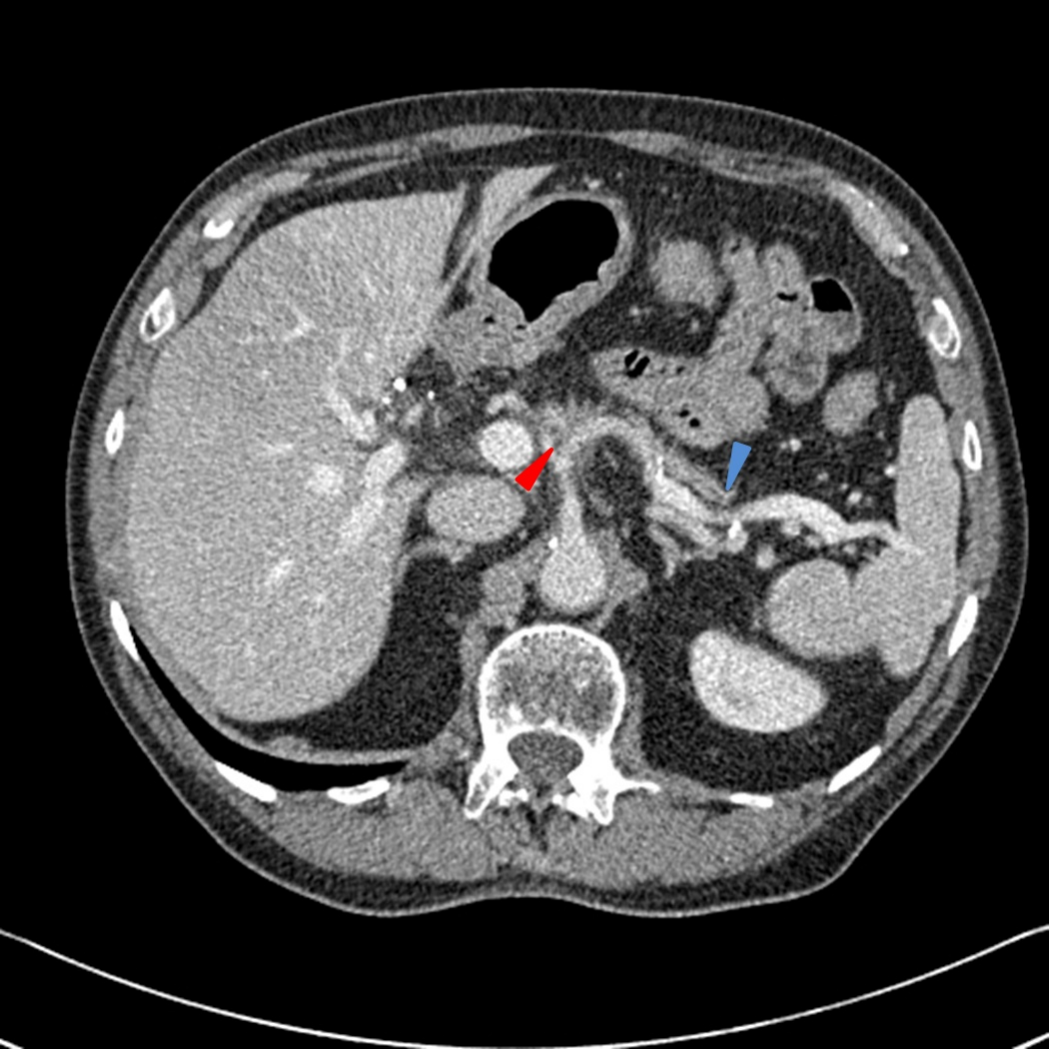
CT scan of the abdomen and pelvis obtained greater than 36 months after diagnosis There is pancreatic atrophy (blue arrow) with stable-appearing soft tissue surrounding the celiac axis (red arrow). There are stable-appearing peripancreatic lymph nodes and the previously seen peripheral hepatic hemangioma is no longer visible. The portal and hepatic vessels appear patent.
